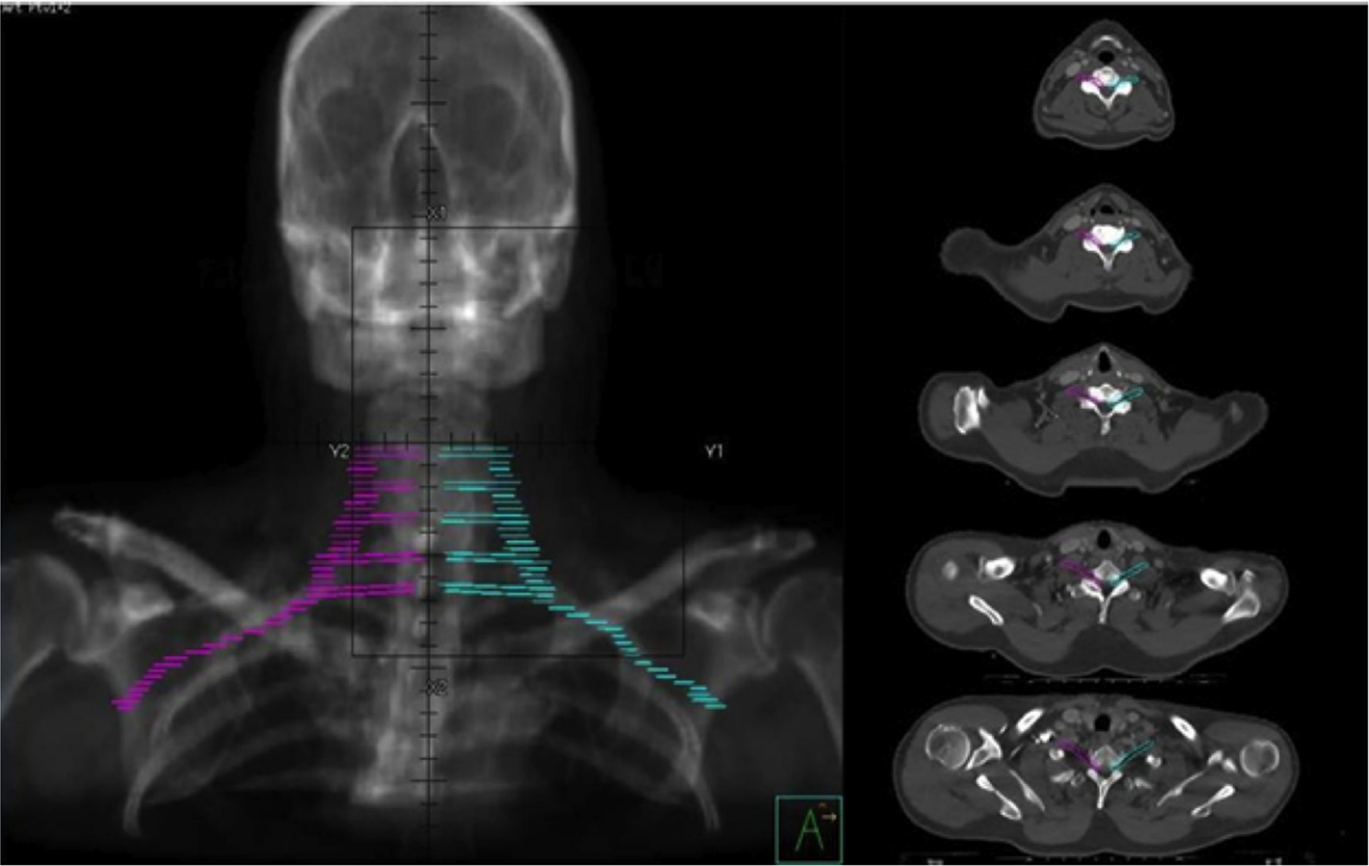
Digital reconstructed radiograph of contoured brachial plexus with the right BP in magenta and left BP in cyan. Right panel shows axial images of representative slices contoured with the right and left BP.
